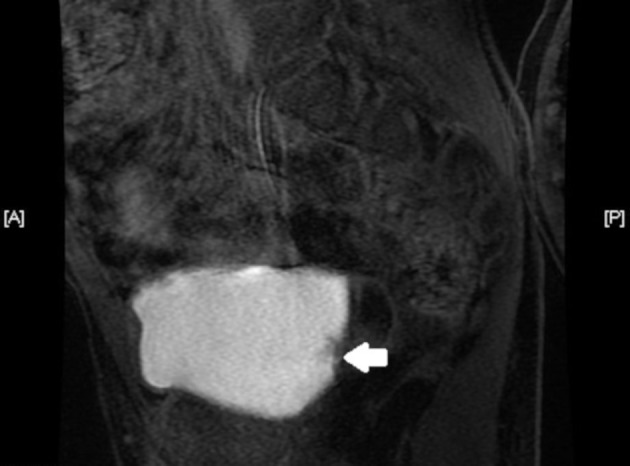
Abdominal MRI, in axial section, showing the lesion (indicated by the white arrow) restricted to the bladder mucosa, close to the right ureteral ostium.
